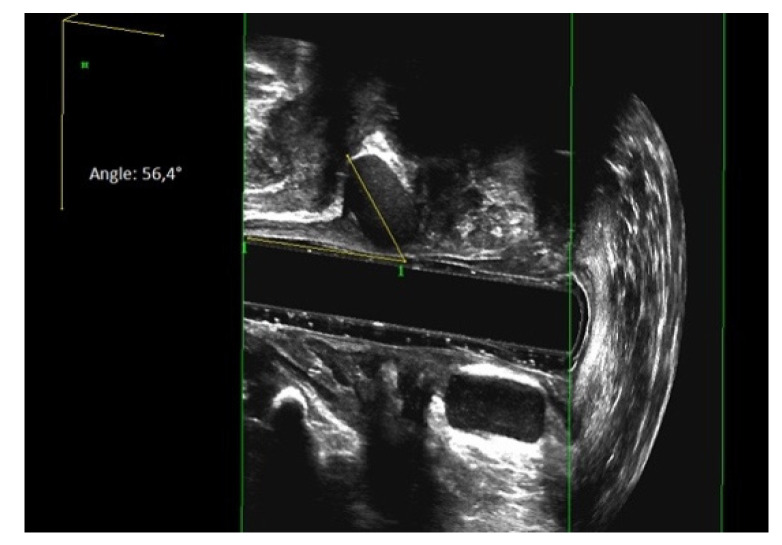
3D-endoanal ultrasound image: A migrated prosthesis in a 56.4° angle to the anal canal.
